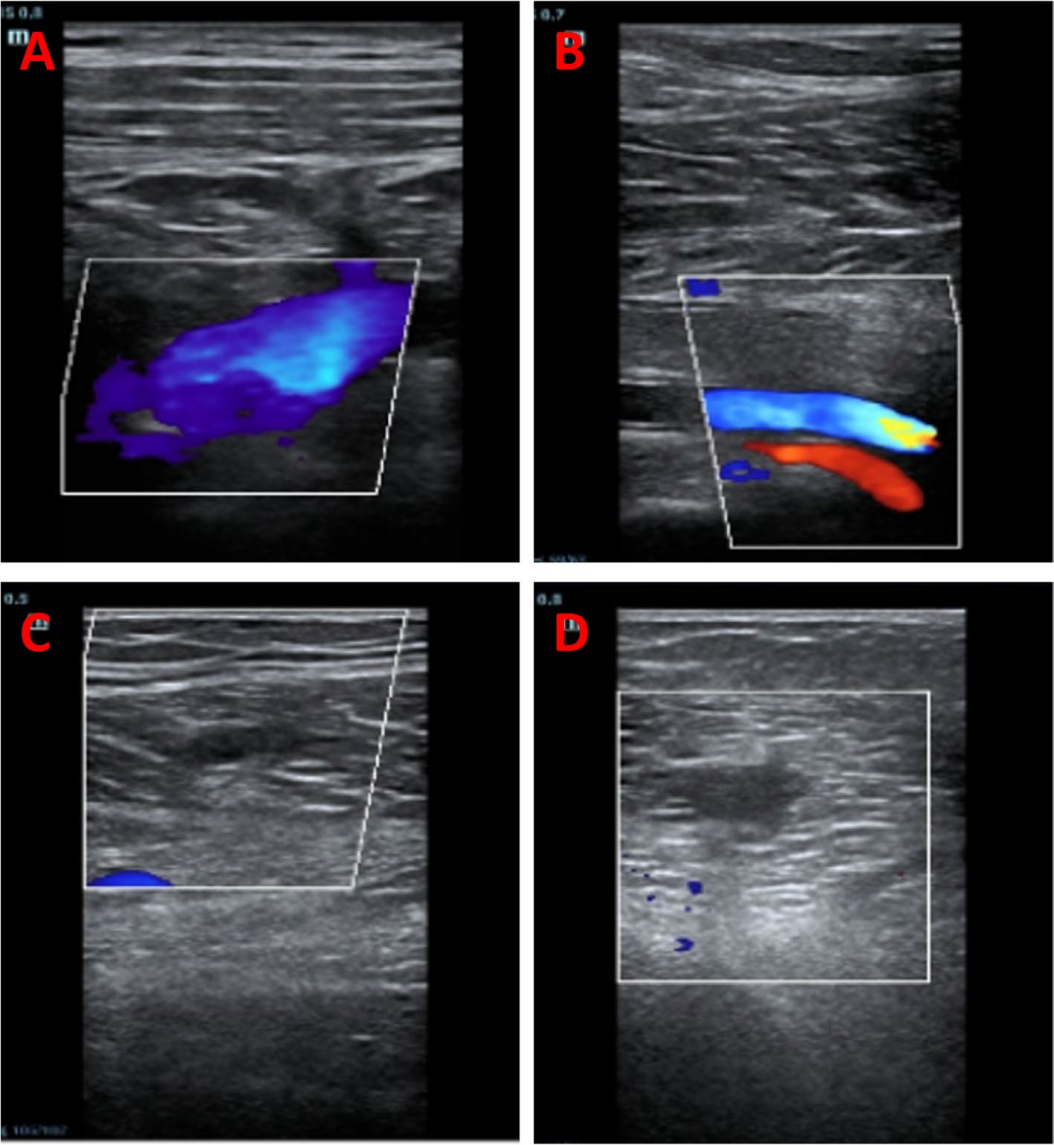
Ultrasound of limbs and internal jugular vein on 14th May. A left subclavian vein; B left popliteal vein; C left intermuscular calf vein; D Right intermuscular calf vein
